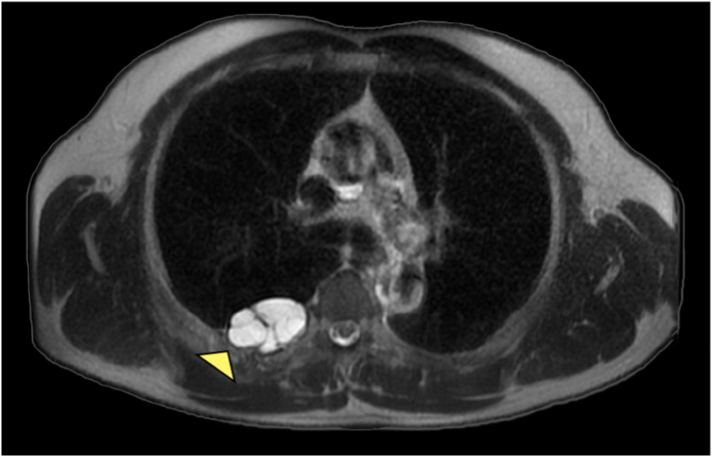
Axial T2-weighted MRI showing a cystic lesion with a daughter cyst measuring 46 mm in total located at the sixth rib's posterior arch.
